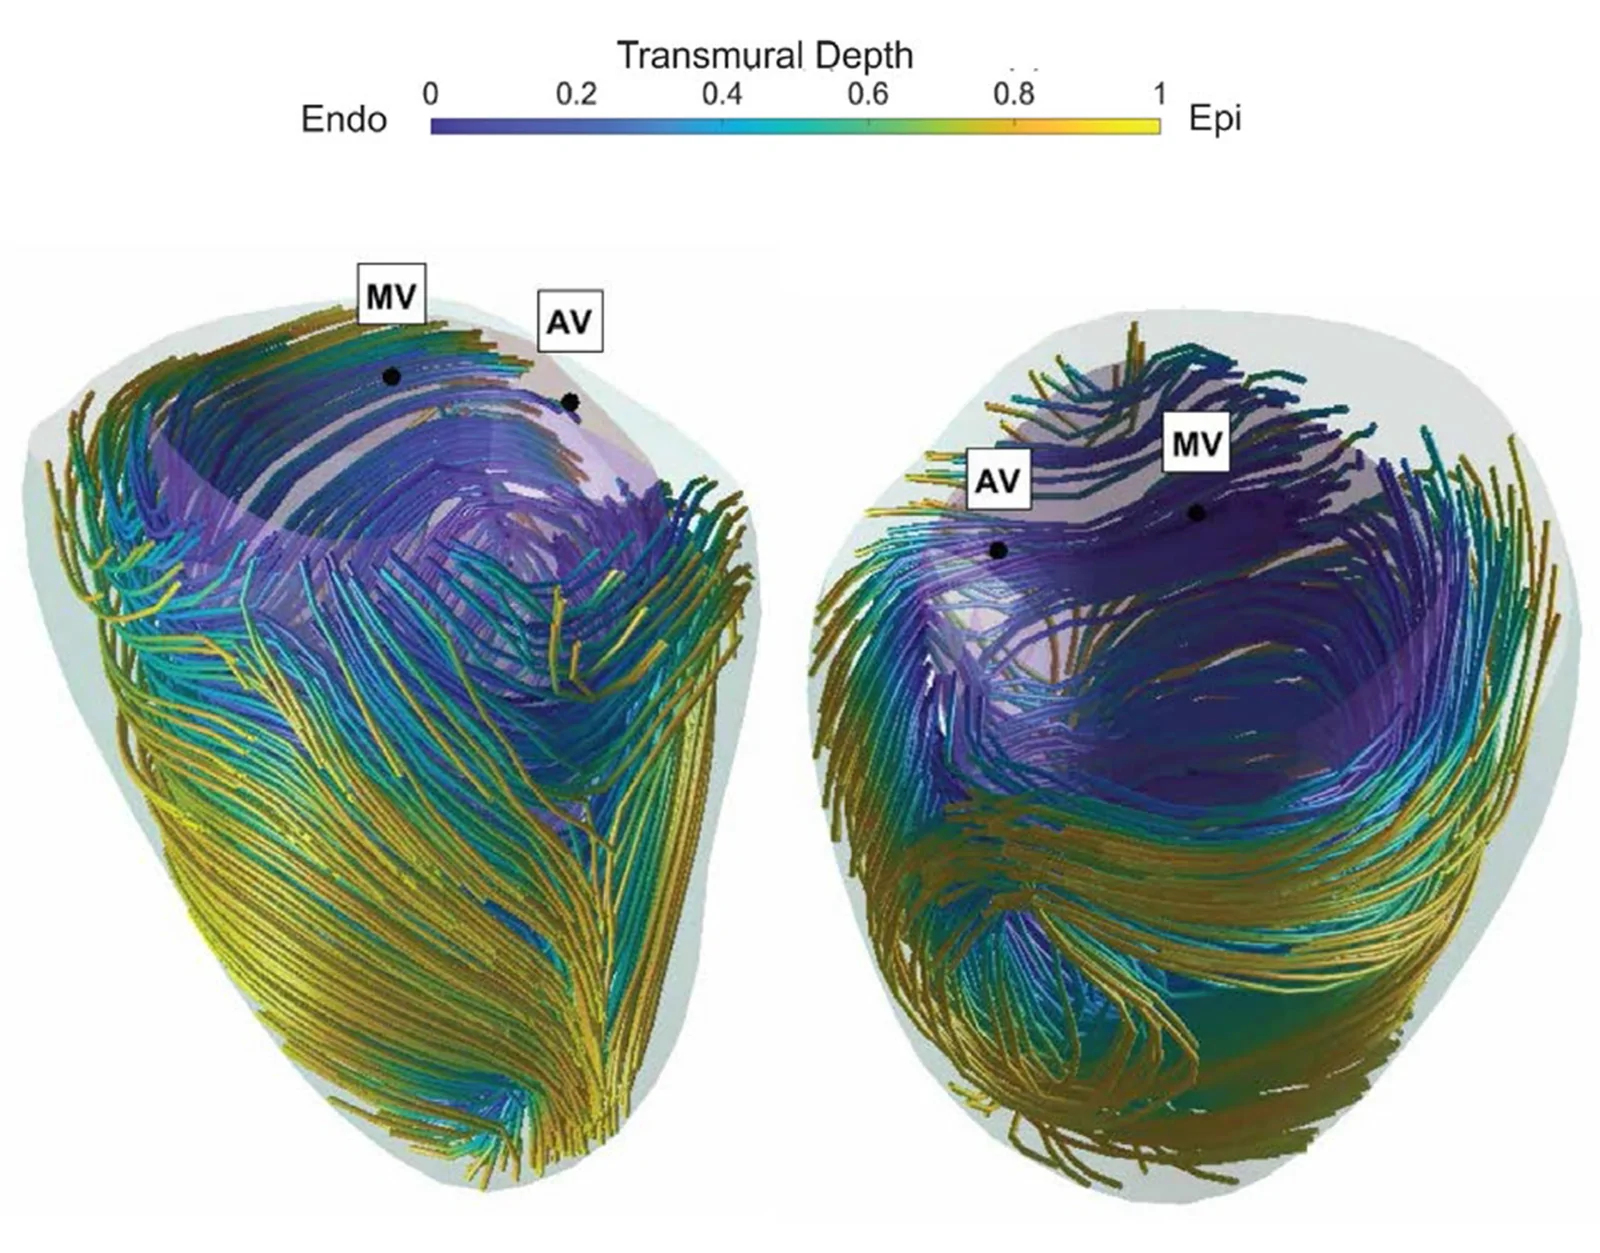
Principal strain analysis derived from 3D echocardiography. The strain lines indicate the direction of maximum contraction and are color-coded by the depth (0 on the endocardium and 1 on the epicardium). The results shown in the figure correspond to the healthy left ventricle (LV). The mitral and aortic valves are labeled as MV and AV, respectively, to aid spatial orientation
